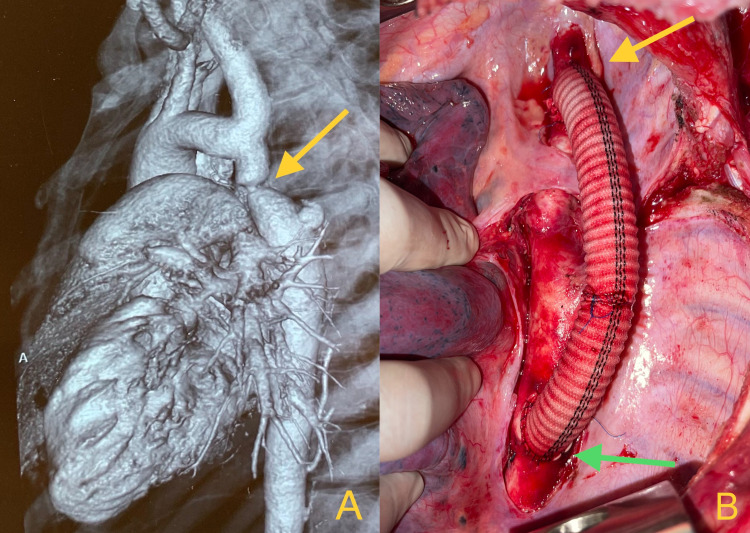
(A) CT volume rendered image (CRT) showing post subclavian coarctation of aorta with post stenotic dilatation of aorta (yellow arrow); (B) Intraoperative picture showing left subclavian artery (yellow arrow) to descending thoracic aorta bypass (green arrow) with polyester graft.
